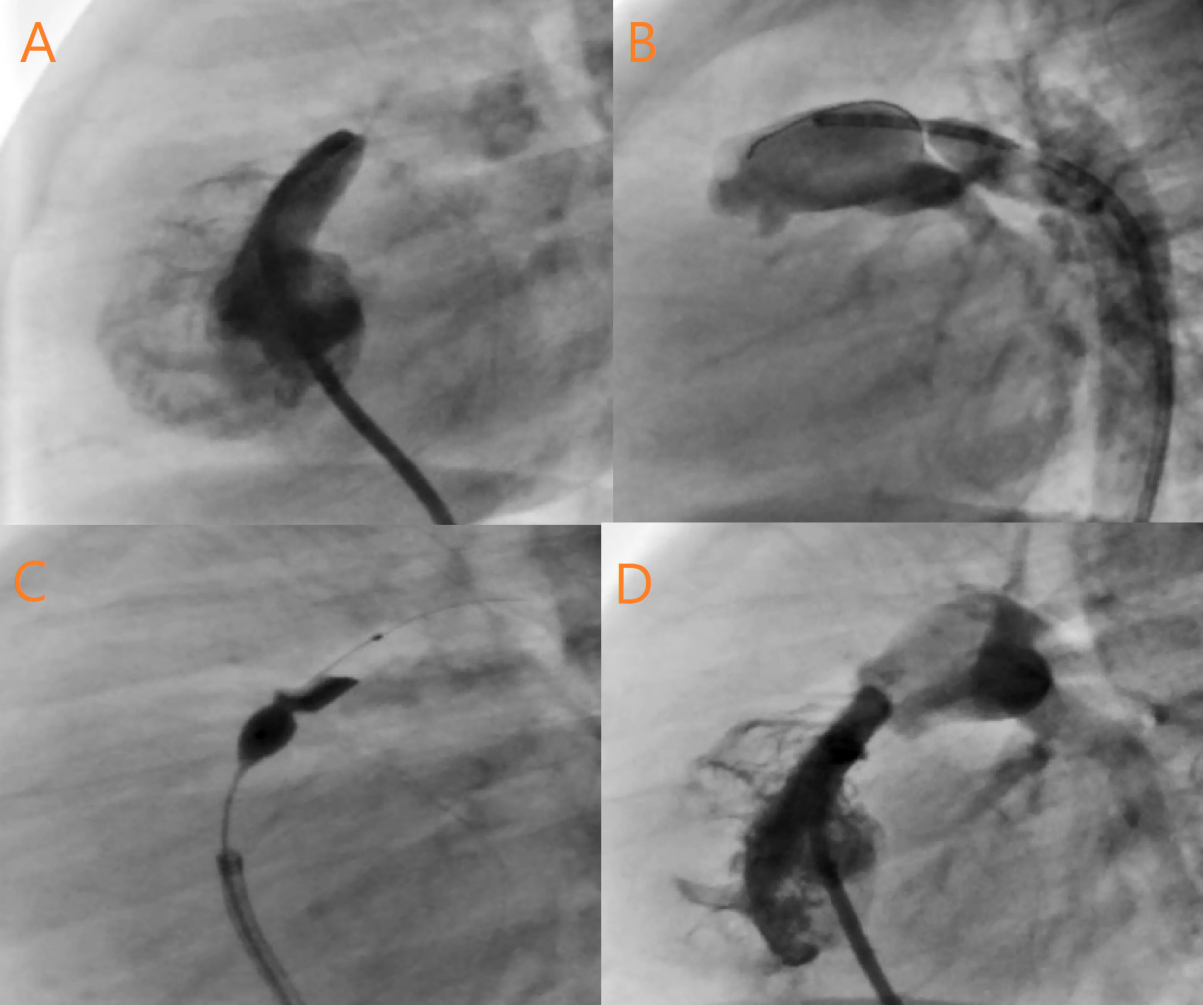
The selection of the appropriate balloon size was based on the echocardiography and catheterization measurements. The diameter of the pulmonary valve annulus was measured in the short-axis view via echocardiography. During the cardiac catheterization, the diameter of the pulmonary annulus was calculated in the anteroposterior and lateral views after the injection of the contrast medium into the RV.
